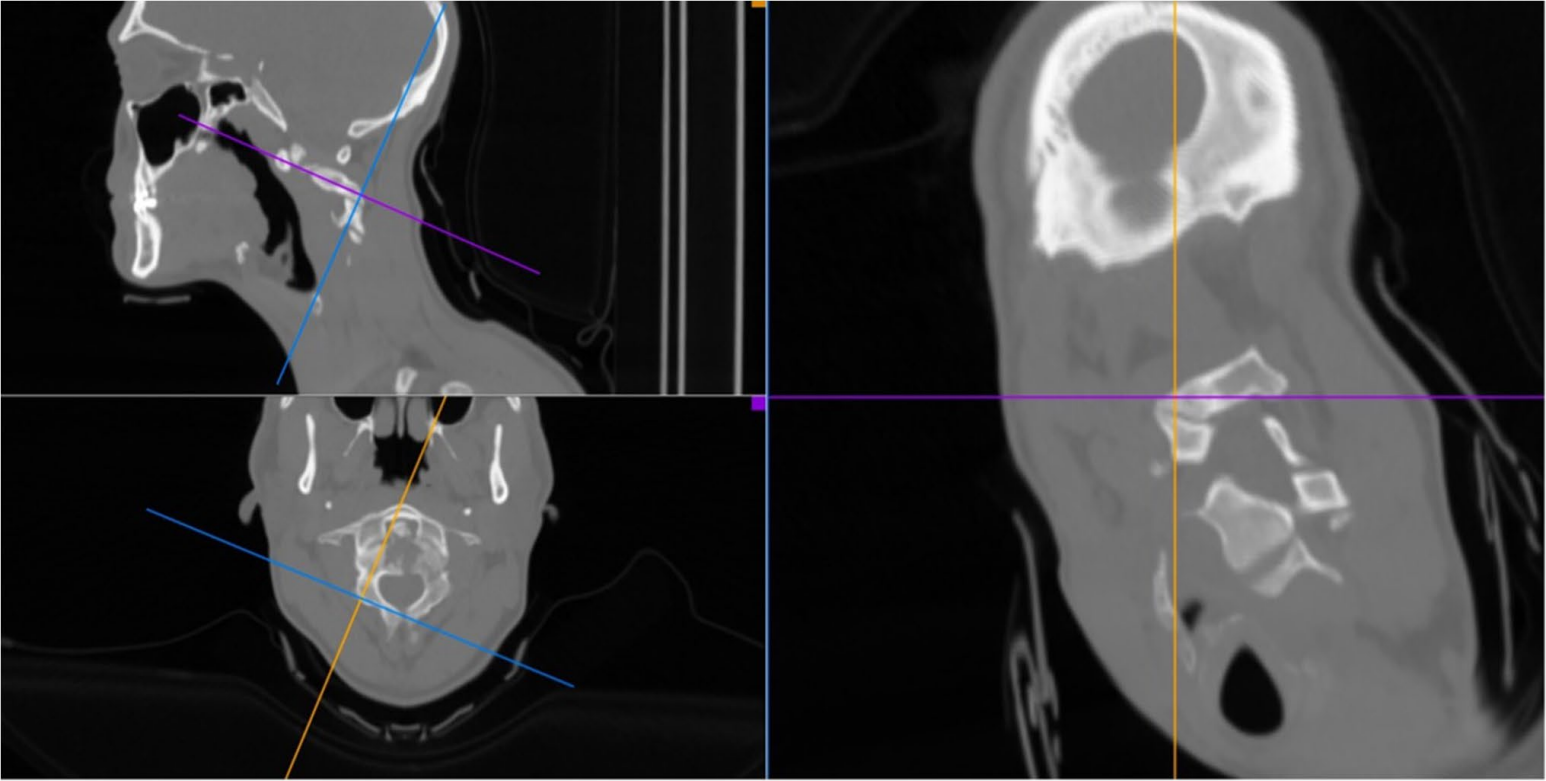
CT scan MPR reconstruction for planning screws trajectory using the open-source software Horos™
